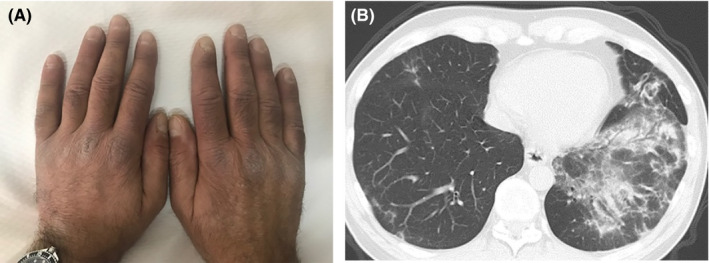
A, Flat violaceous papules on the dorsum of the metacarpophalangeal joints. B, High‐resolution computed tomography disclosed ground glass opacities likely consistent with organizing pneumonia
